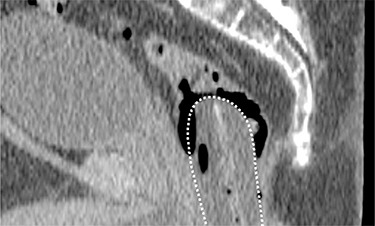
Sagittal CT image of a patient with a large amount of rectal gas. The rectal balloon is delineated by the white dotted line. Gas is trapped and has accumulated between the endorectal balloon and the rectal wall, distending the rectum and possibly displacing the prostate. Air bubbles are also present within the rectal balloon.
